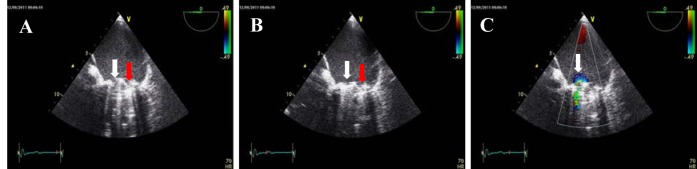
Transoesophageal echocardiography showing reduced mobility of the prosthetic valve with functional stenosis. (A) Diastole. (B) Systole. (C) Colour Doppler of the diastole. The white and red arrows point to the mobile and immobile leaflets, respectively.
